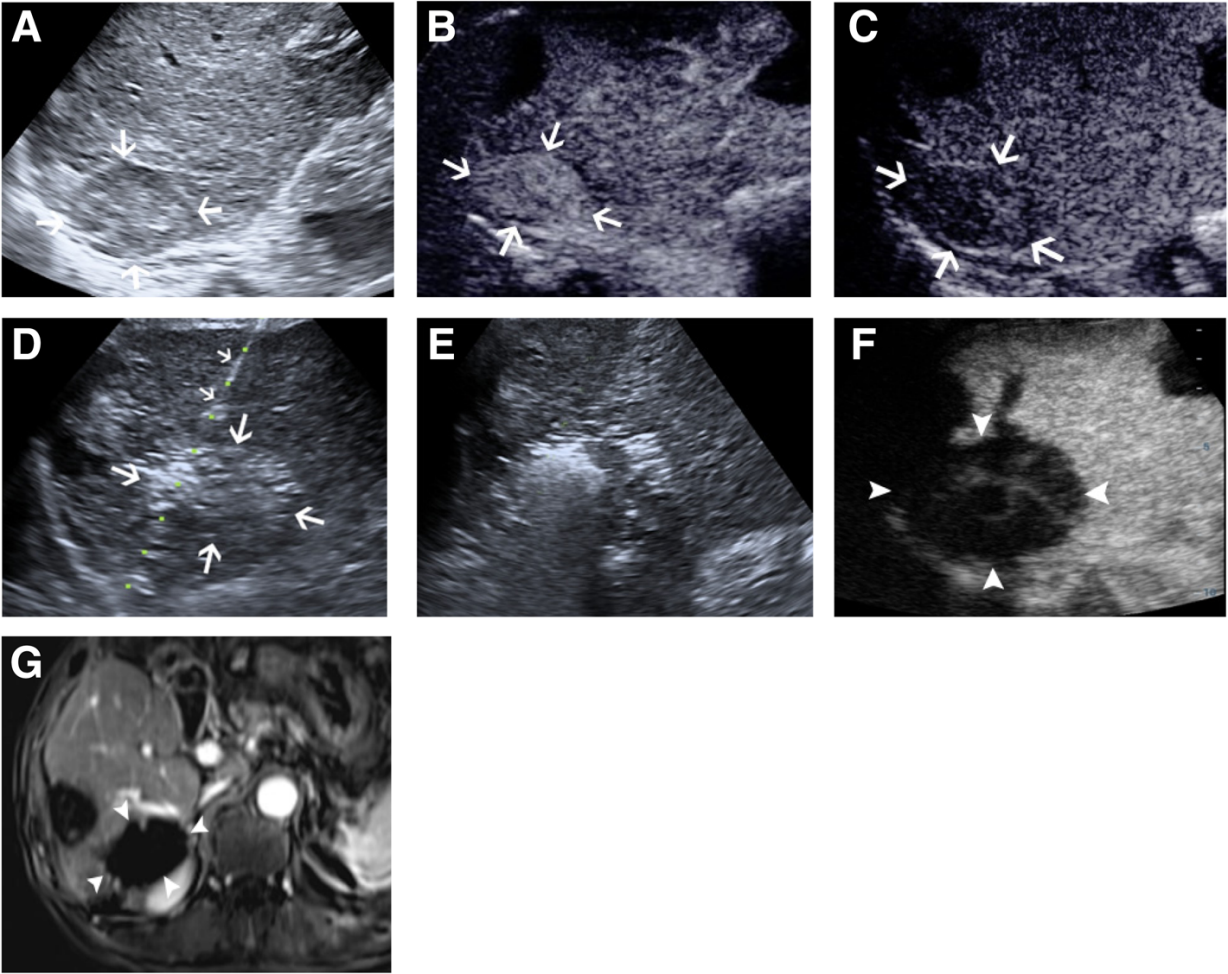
Radiofrequency ablation (RFA) in a 66-year-old man with metastatic hepatocellular carcinoma in the right adrenal gland. a Pre-interventional B-mode ultrasound showed a round mass at the upper pole of the right kidney (arrow). b and c contrast-enhanced ultrasound (CEUS) before RFA showed obvious enhancement in the early phase (b) and washout in the late phase (c). d Prober placement during the ablation of the right adrenal metastasis. e Tumour appeared completely hyperechoic after the beginning of RFA. f Follow-up CEUS 1 month after RFA showed no contrast enhancement, suggesting complete ablation. g Follow-up MRI 1 month after RFA showed no enhancement of the target tumour
